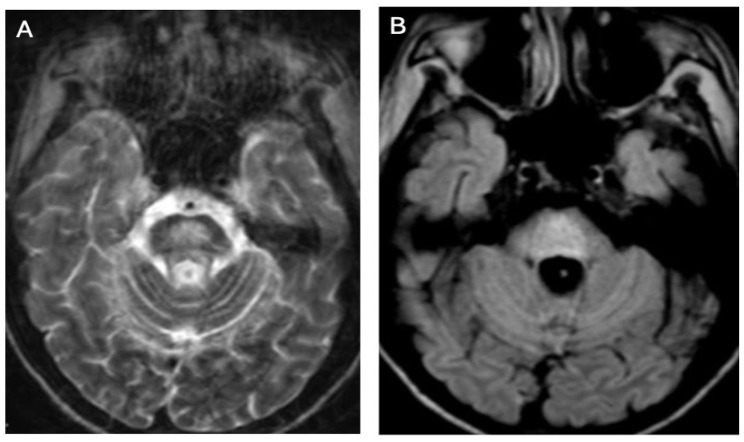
Hyperintense changes localized in pons in T2-weighted images (A) and FLAIR (B) (own materials of the neurology department).
